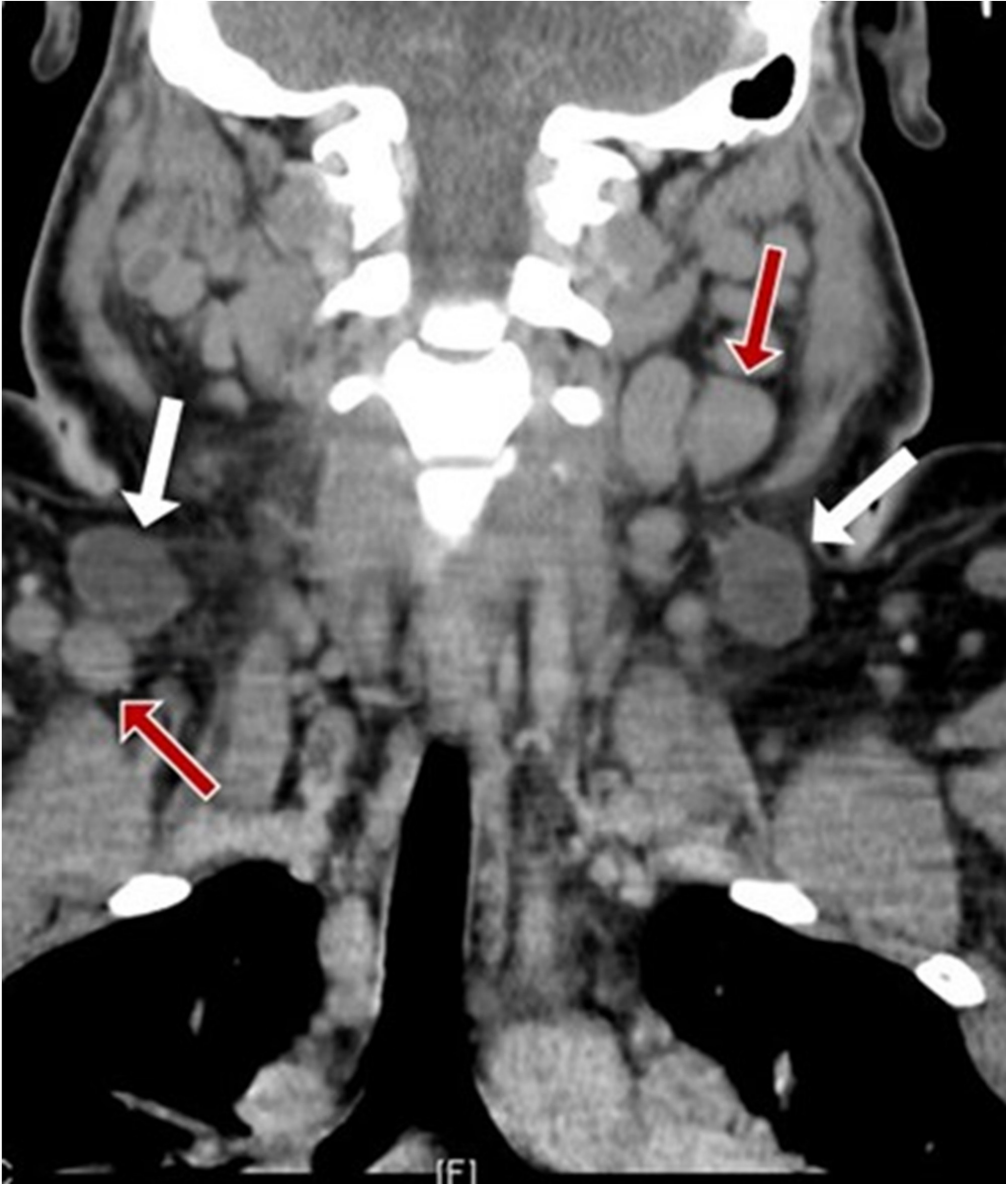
Computed tomography (CT) of the neck and chest. Marked cervical, supraclavicular, mediastinal, and upper abdominal lymphadenopathy and hepatosplenomegaly. Centrally necrotic cervical lymph nodes (white arrows) are compared to enlarged, but non-necrotic, lymph nodes (red arrows).
